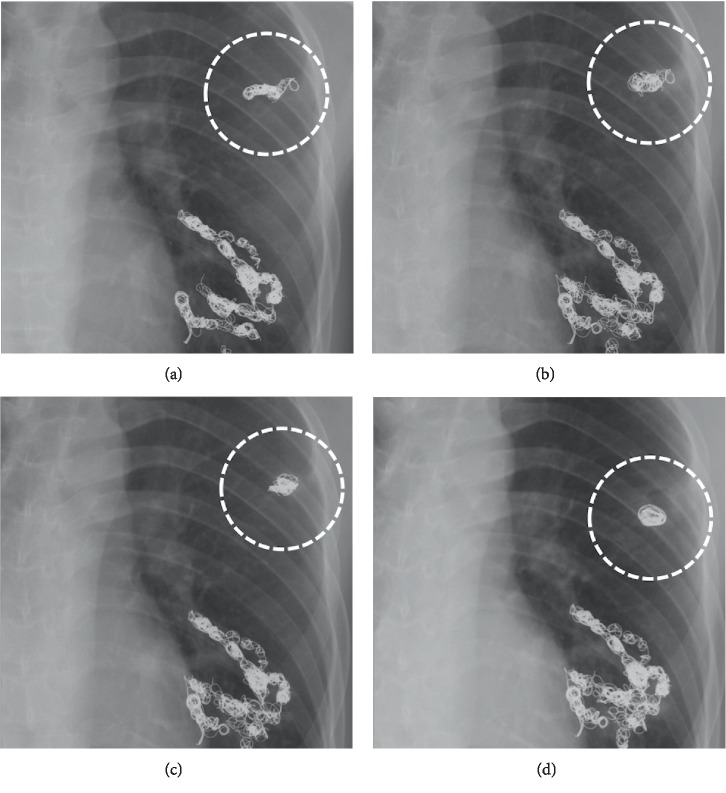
Chest radiograph images during follow-up showed that the coils used in embolization of the pulmonary arteriovenous malformation of the left upper lobe became deformed. (a) 11 years before, (b) 4 years before, (c) 2 years before, (d) latest examination.
